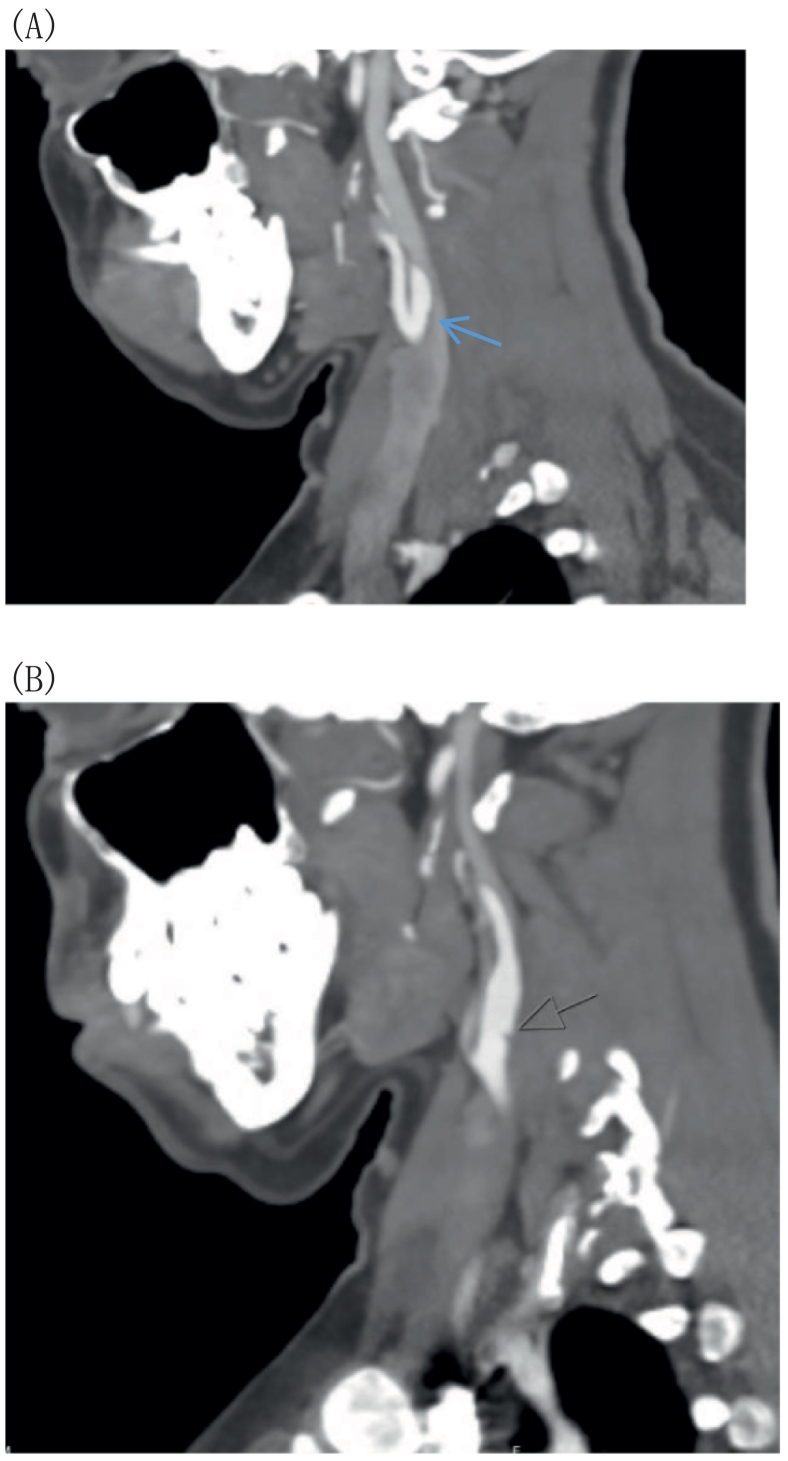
A and B: The sagittal view CT angiogram of the neck showed a shelf-like filling defect in the proximal internal carotid artery, with a blue arrow indicating the right ICA and a black arrow indicating the left ICA. (For interpretation of the references to colour in this figure legend, the reader is referred to the web version of this article.)
